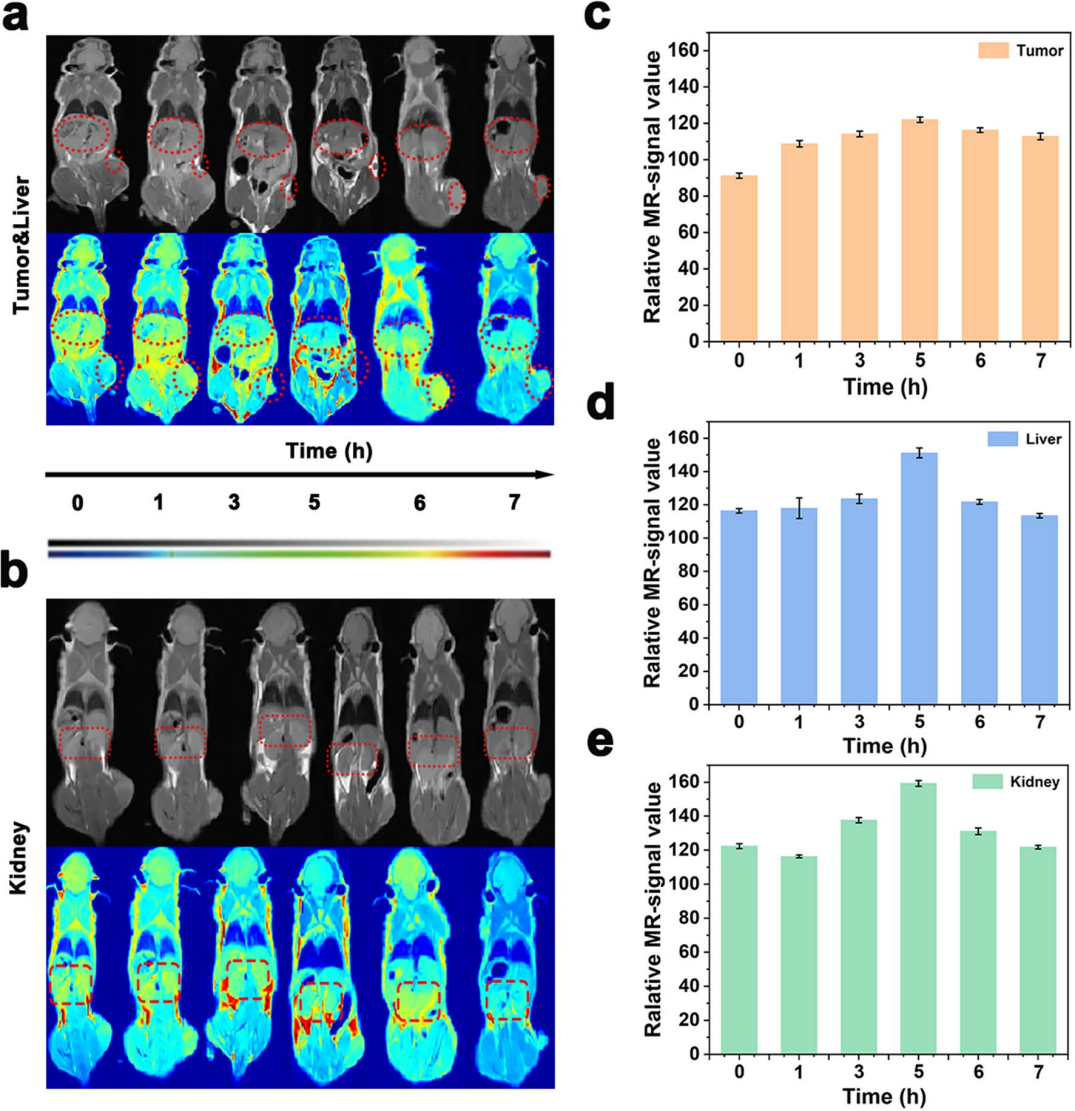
MRI images of the HCF@β-lap in vivo. a MRI images of the tumor (indicated with small ellipses) and liver (indicated with large ellipses). b MRI images of the kidney (indicated with rectangles). c–e Corresponding MRI signal intensities for the images shown in a, b
